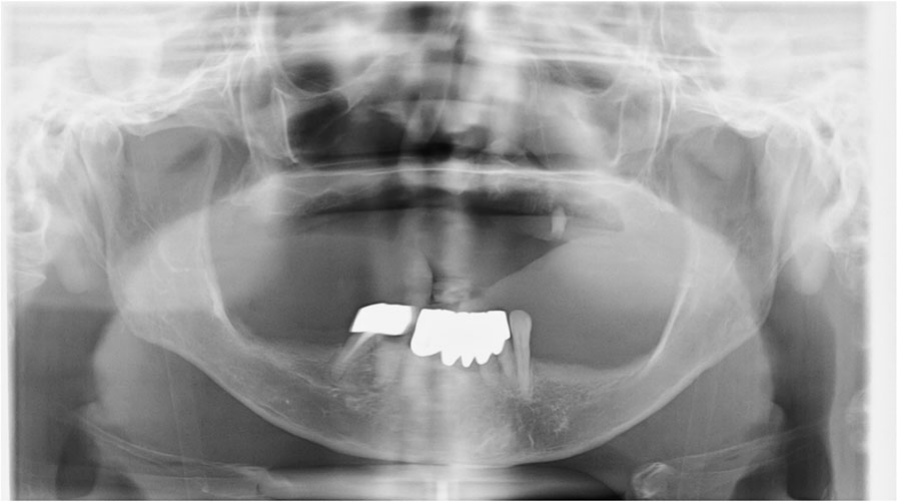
Panoramic radiograph. A transparent image is seen in the apical region of tooth No. 25
